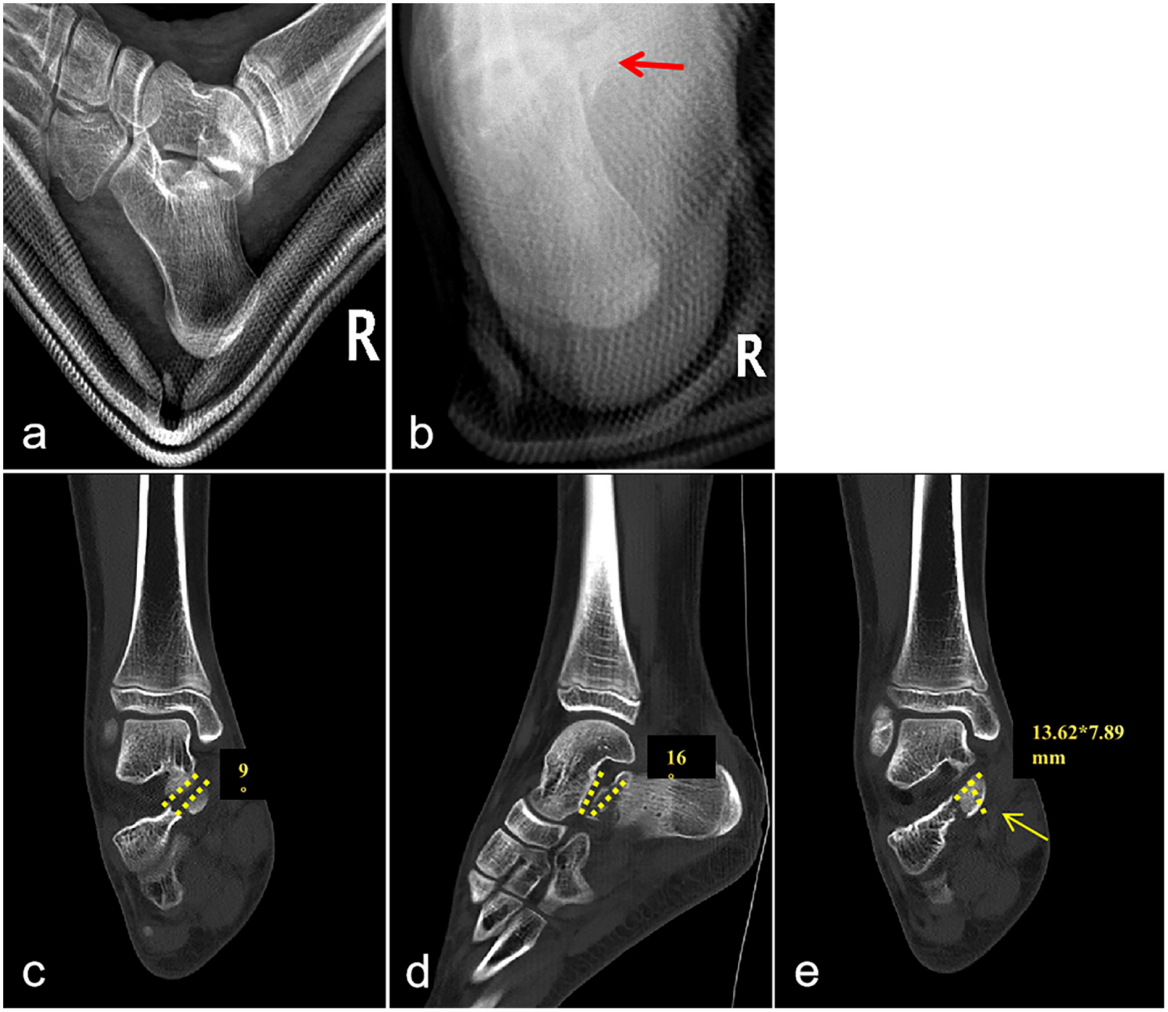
Preoperative x-ray and CT images. (a) Lateral view of the calcaneus reveals no clear fracture line. (b) Axial view demonstrates a distinct fracture line (red arrow), indicative of a sustentaculum tali fracture, though displacement remains uncertain. (c) Coronal CT image demonstrates articular surface disruption with approximately 9° of angulation. (d) Sagittal CT image reveals an articular surface angulation of approximately 16°. (e) The fracture fragment measures approximately 13.62 mm × 7.89 mm.
